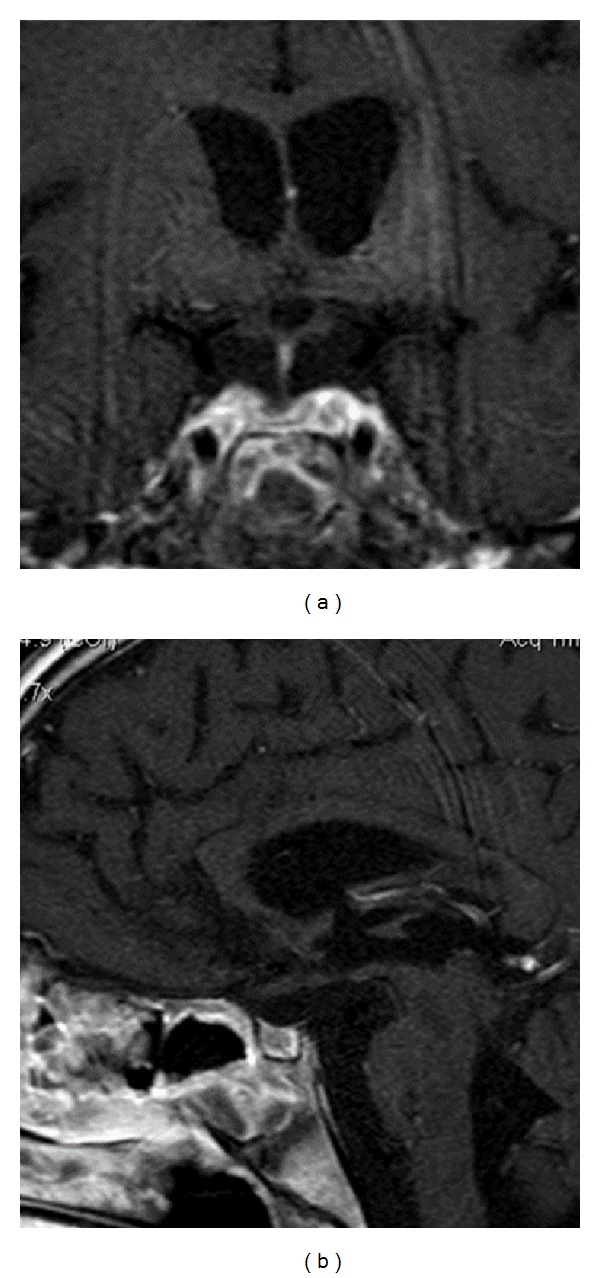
Axial T1-weighted (a) and sagittal T1-weighted (b) MRI after gadolinium enhancement one month after chemotherapy, MRI showed resolution of pituitary-hypothalamic mass, with a marked reduction in stalk-thickness.
